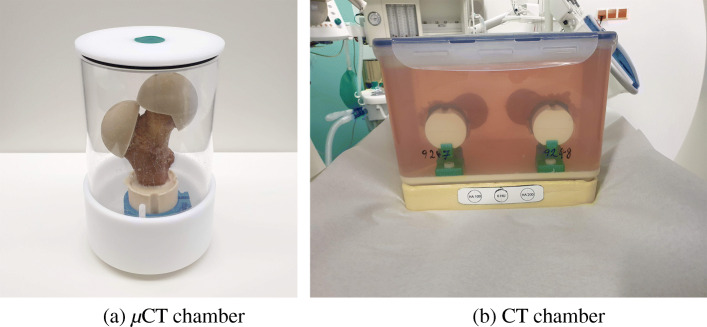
a) *μ*CT chamber from POM and Plexiglass to fix the sample in neutral alignment and keep it hydrated throughout the scanning process, b) CT chamber from polypropylene plastic fitted with embedding base, and holders to accommodate a pair of samples submerged in saline solution and in neutral alignment
